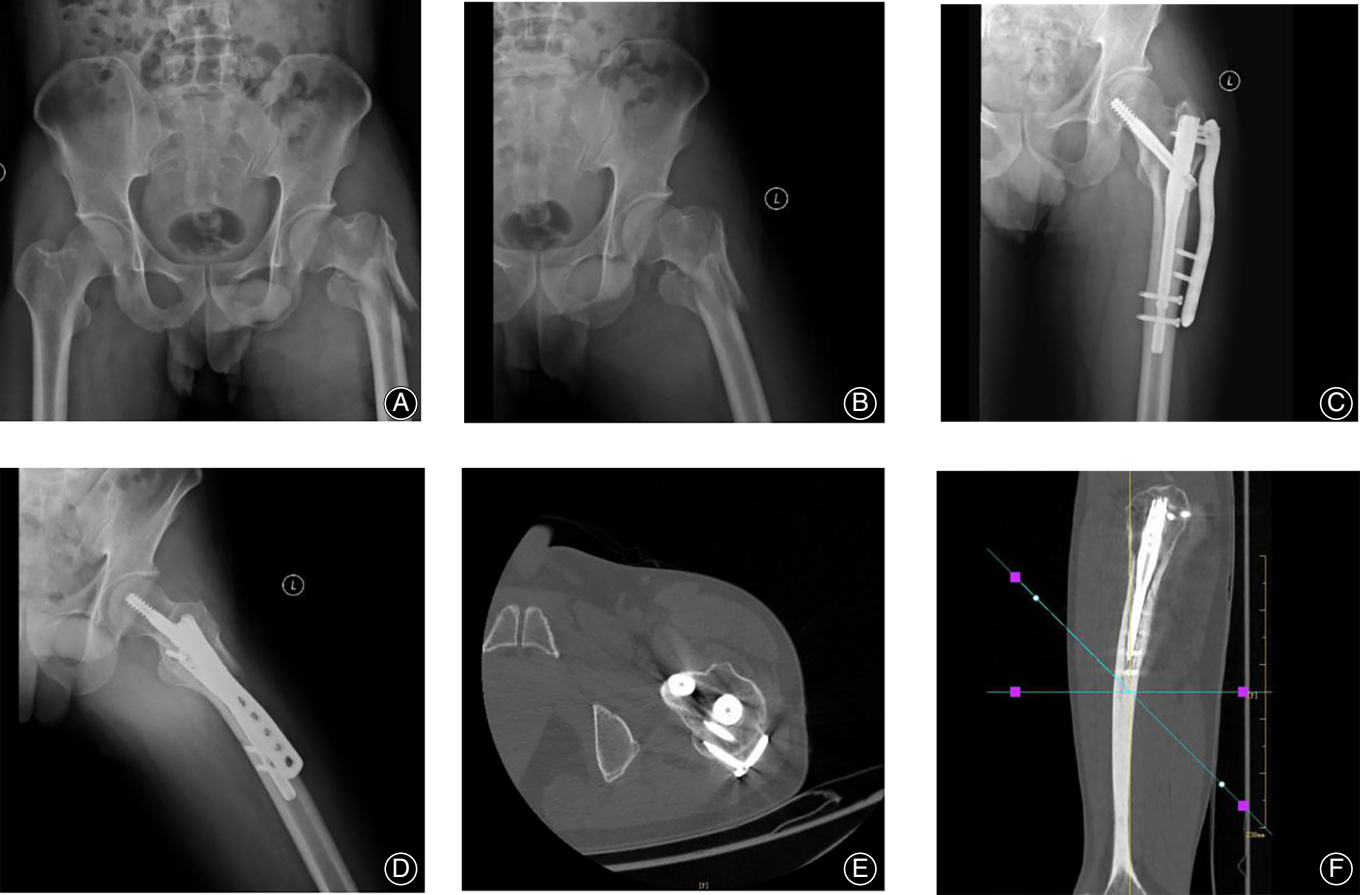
A 57‐year‐old male patient with trochanteric fracture was treated with intramedullary nail assisted anatomical locking plate fixation of the lateral wall of the proximal femur. (A, B): X‐ray lateral wall injury was serious before operation. (C, D): After operation, X‐ray positive and lateral films showed anatomical reduction and reliable fixation of fracture. (E, F): 6 months after operation, CT showed bone healing.
